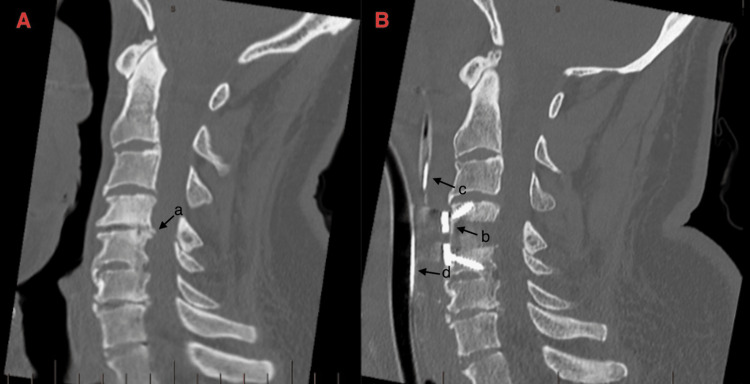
Pre-operative and post-operative computed tomography scans. (A) Pre-operative computed tomography (CT) scan of the cervical spine demonstrating multilevel degenerative changes. (a) At the C4/5 level, there is a disc osteophytic bulge, with posterior osteophytic protrusion, causing mild to moderate impingement on the anterior chord. (B) Post-operative CT. (b) C4/5 anterior discectomy and fusion after successful haematoma evacuation. Anatomical alignment is maintained, with degenerative changes in the adjacent levels. (c) Nasogastric tube. (d) Tracheal tube.
